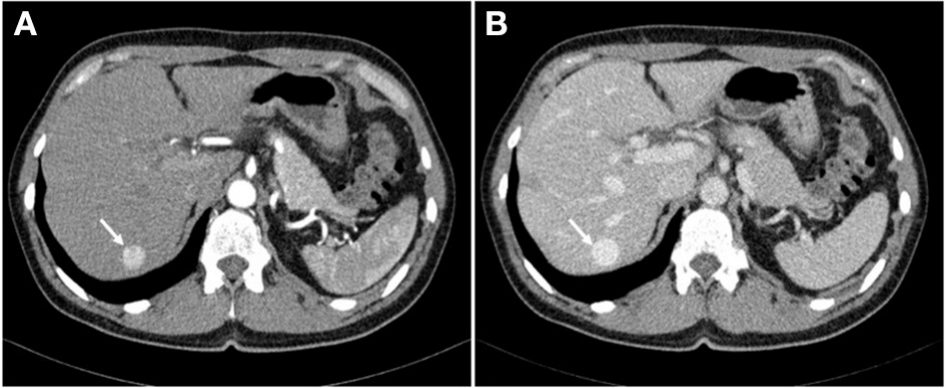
Abdominal CT scan demonstrating a 15 mm hypervascular lesion in segment VII of the liver in arterial phase (A) and venous phase (B).
